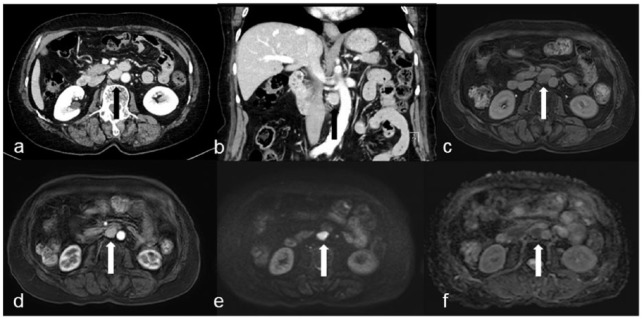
Abdominal CT shows a well-enhanced 25-mm retroperitoneal tumor that is located between the aorta and IVC (arrow): (a) axial CT image, (b) coronal CT image. MRI shows that the tumor is a high signal intensity on (c) T2-weighted image, (d) a well enhanced on T1-weighted image, (e) high signal intensity on diffusion-weighted image, and (f) low signal intensity on ADC-map.
